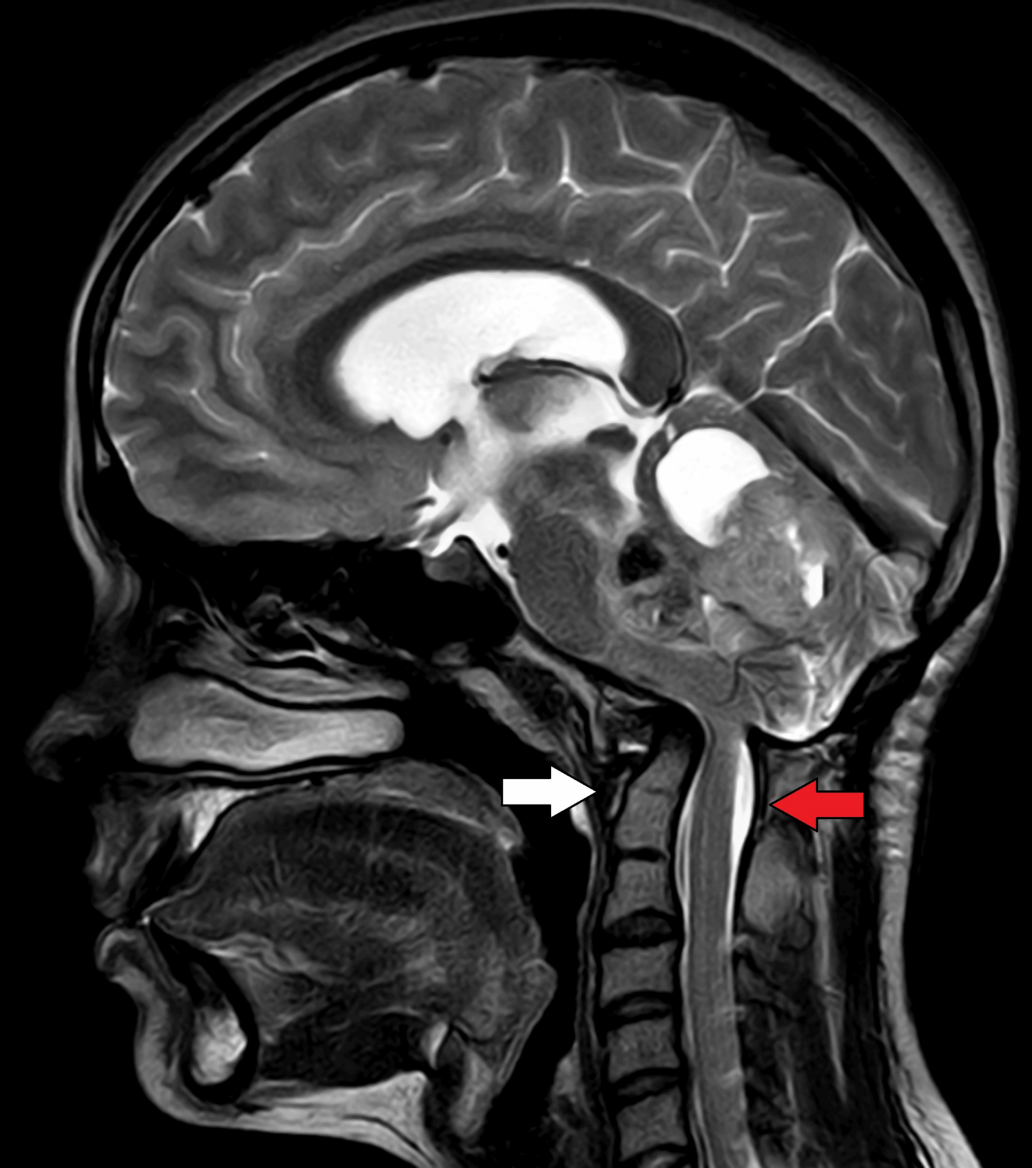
Sagittal MRI brain image The red arrow shows partial assimilation of the occipital bone with C1 vertebra, cervical-medullary kinking with no evidence of oedema. The white arrow shows narrowing is noted at the level of the foramen of magnum with crowding within due to basilar invagination. MRI: Magnetic resonance imaging
